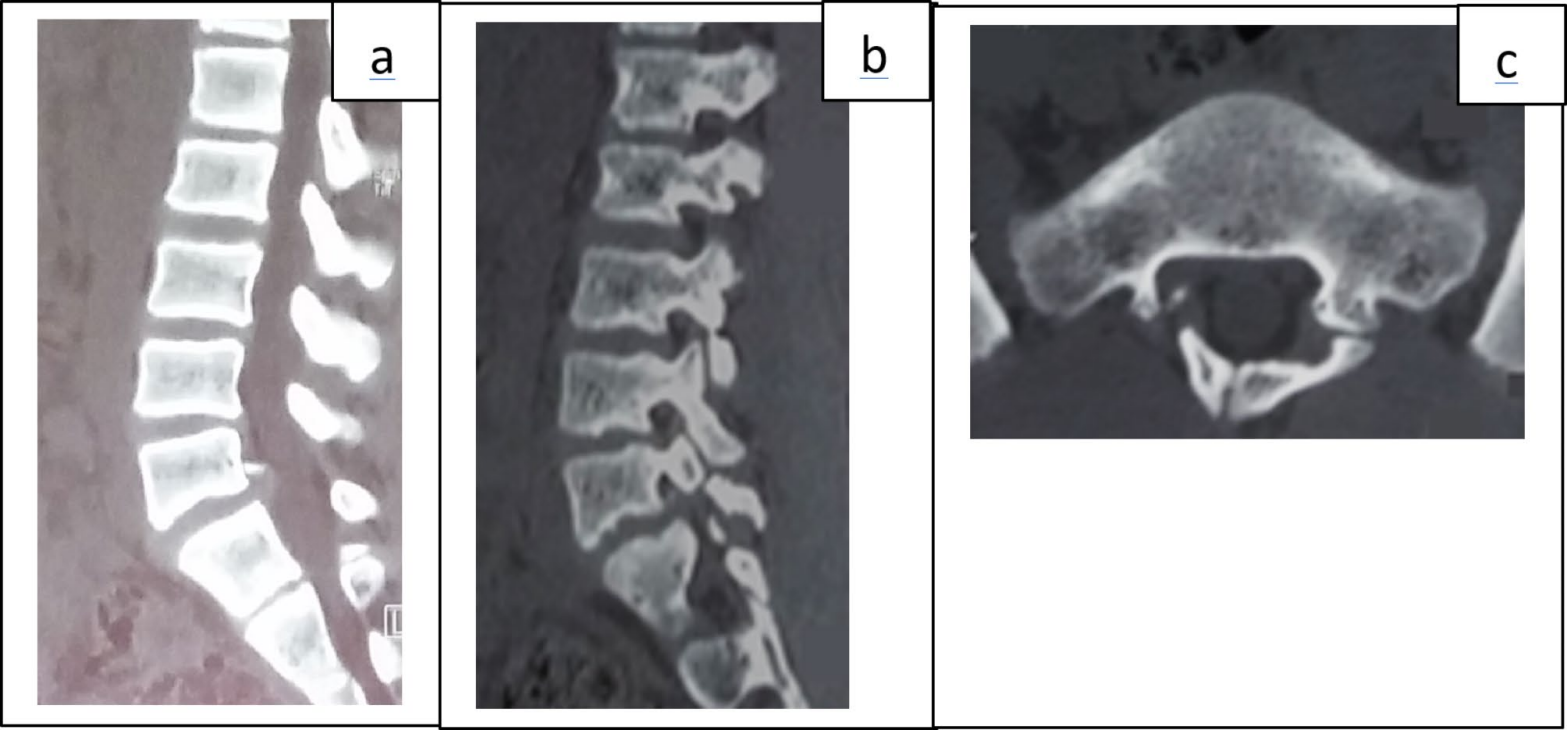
CT images of the patient of the observation 2. CT in sagittal reconstruction, parenchymal window, passing through the spinal canal (a) showing a calcified L5S1 disc herniation in its upper part; CT in sagittal reconstruction, bony window, passing through the posterior articular (b) visualizing an undisplaced isthmic lysis of L5; CT in axial section, bony window passing through S1 showing an anomaly of closure of the posterior arch of S1 (spina bifida occulta) (c)
